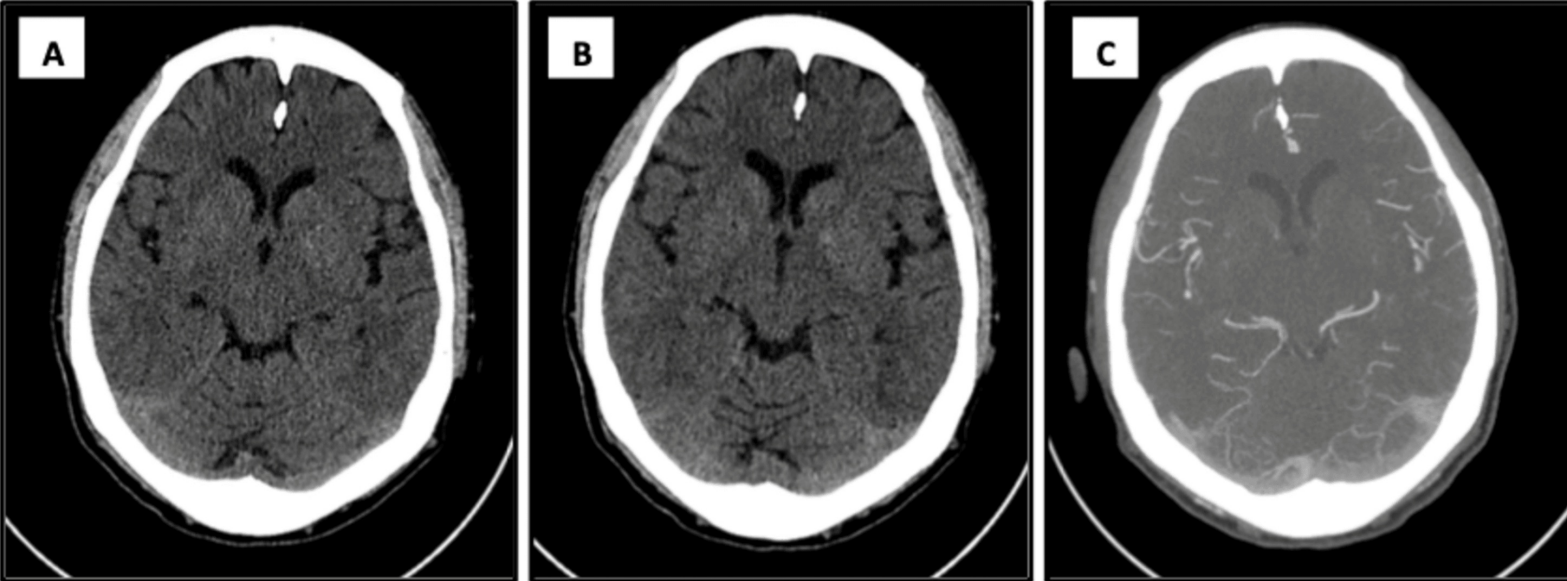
CT imaging of the brain CT without contrast (A and B) demonstrating hyperattenuation in the posterior occipital region.  No acute infarcts or masses or hemorrhages appreciated. CT angiogram of the brain (C) demonstrating similar hyperattenuation due to vasogenic edema. No stenosis or aneurysm of the cerebral vasculature is noted.
